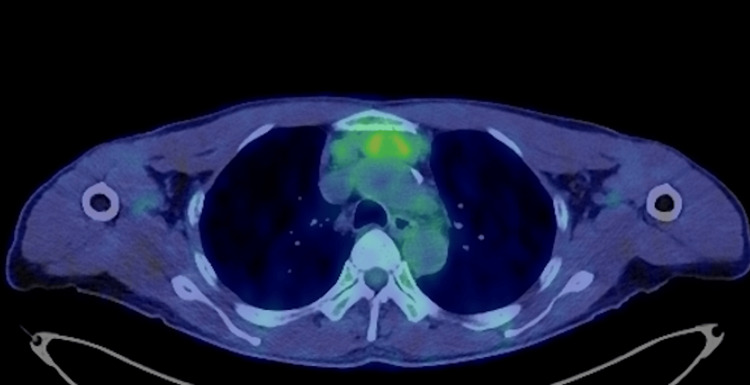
Axial PET/CT image of the thorax showing FDG accumulation This axial PET/CT image of the thorax shows areas of mild abnormal FDG accumulation within the tumor. The SUV max/peak is 3.05/2.51, indicating metabolic activity. Regions with increased FDG uptake are highlighted in green and yellow, suggesting potential pathological conditions such as inflammation or malignancy. FDG, fluorodeoxyglucose; SUV, standardized uptake value
